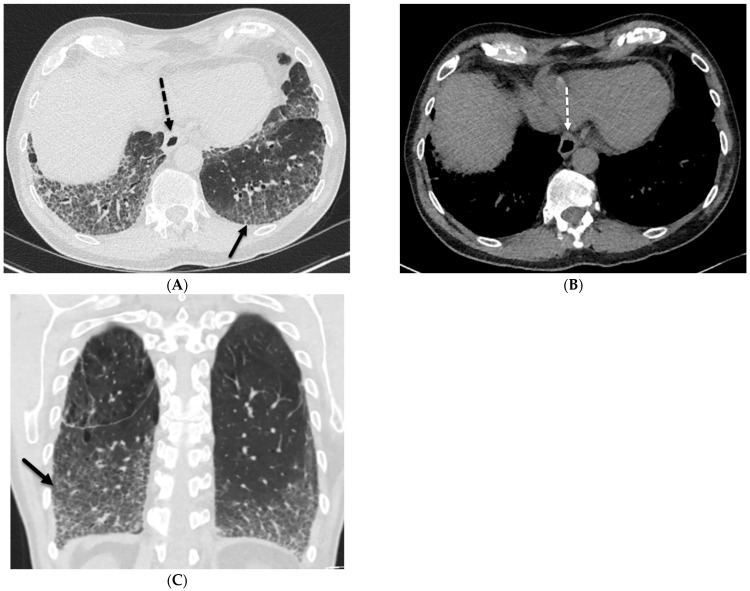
A 62-year-old male with a history of systemic sclerosis. Axial (A,B) and coronal (C) chest CT images demonstrate interstitial fibrotic process characterized by basilar predominant interlobular (solid black arrow) and intralobular septal thickening, traction bronchiectasis, and mild diffuse ground glass opacity without honeycombing. A dilated esophagus (dashed arrow) was also observed consistent with esophageal dysmotility, a common feature of scleroderma.
